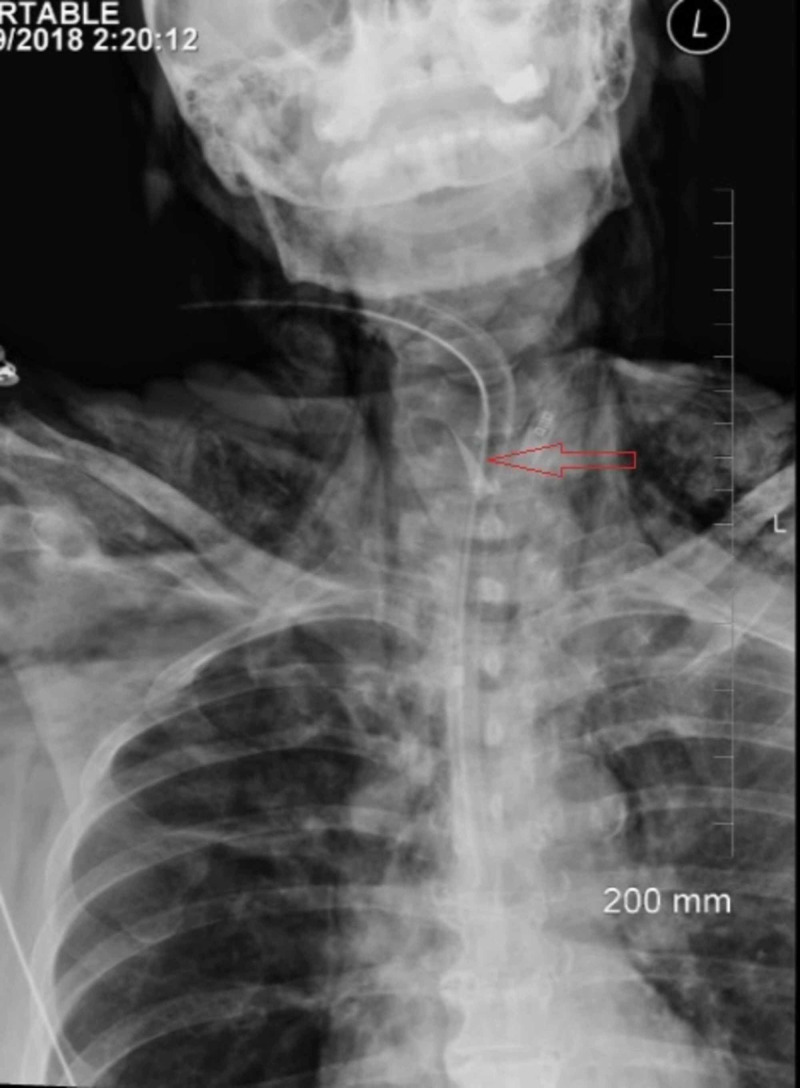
Chest X-ray anteroposterior view showing scalpel blade (labeled)
